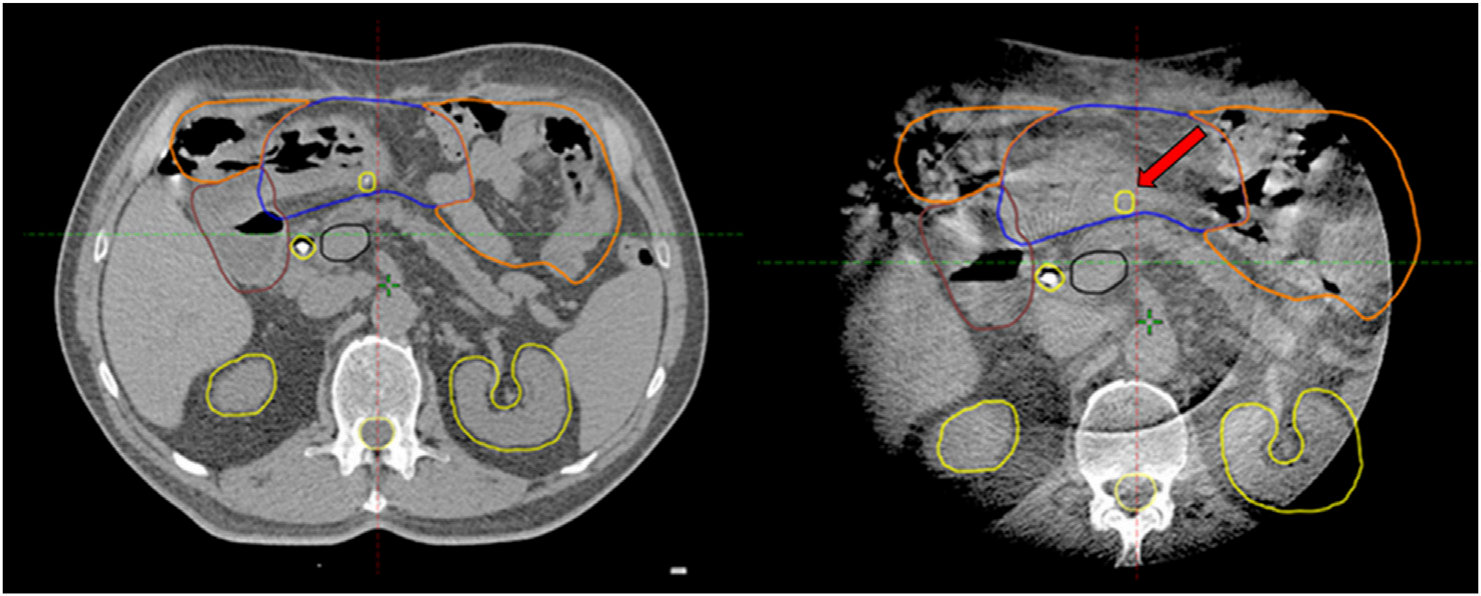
Corresponding axial slices on planning breath-hold-CT and breath-hold-CBCT (half fan mode): Systematic posterior displacement of the stomach (blue) and clip (arrow) was observed during the 1–4th fractions becoming closer to the high-dose area. Consequences/conclusions: 1. The position of this clip (arrow) is no longer relevant during intrafractional triggered imaging 2. This simultaneously alerts radiation therapists to the anatomical changes on CBCT.
